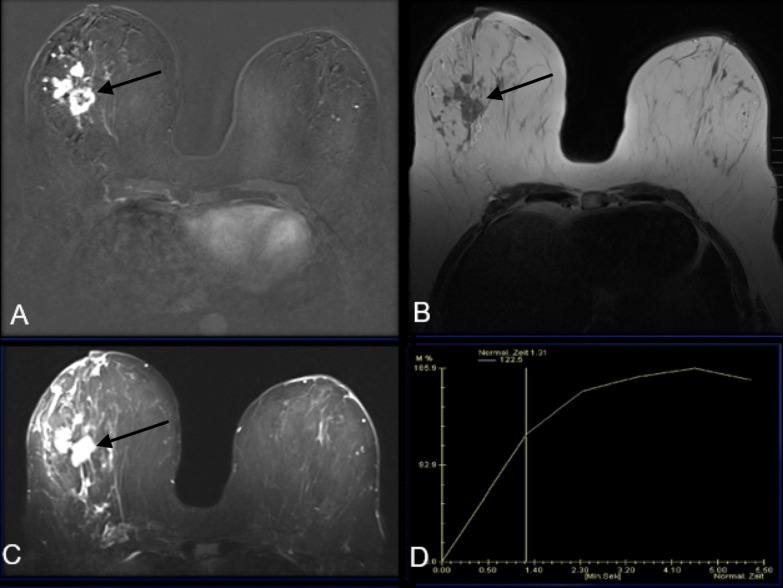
A- MRI subtraction post-contrast image demonstrates an irregular rim
